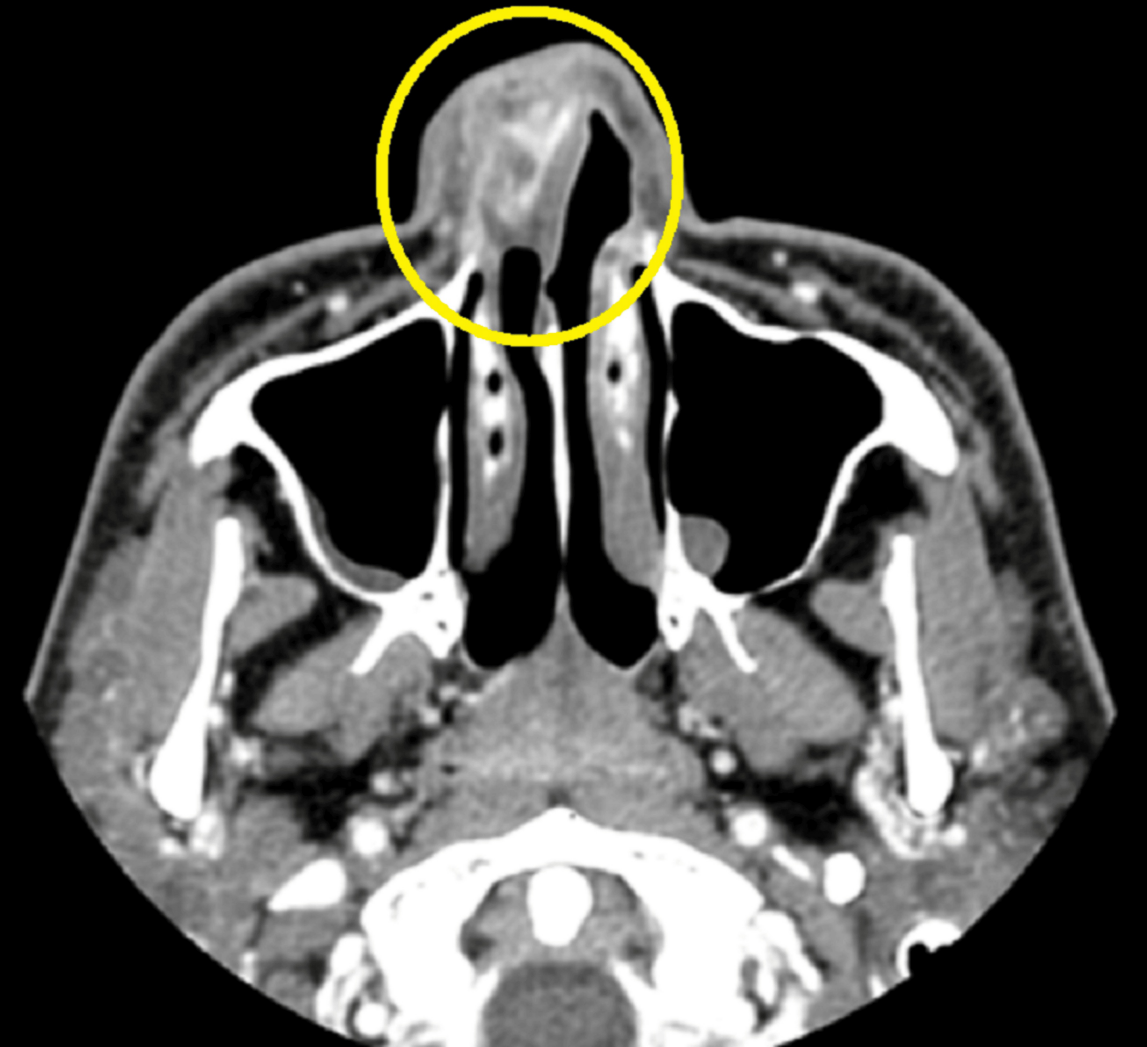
computed tomography. The area enclosed by the circle is the tumor.
